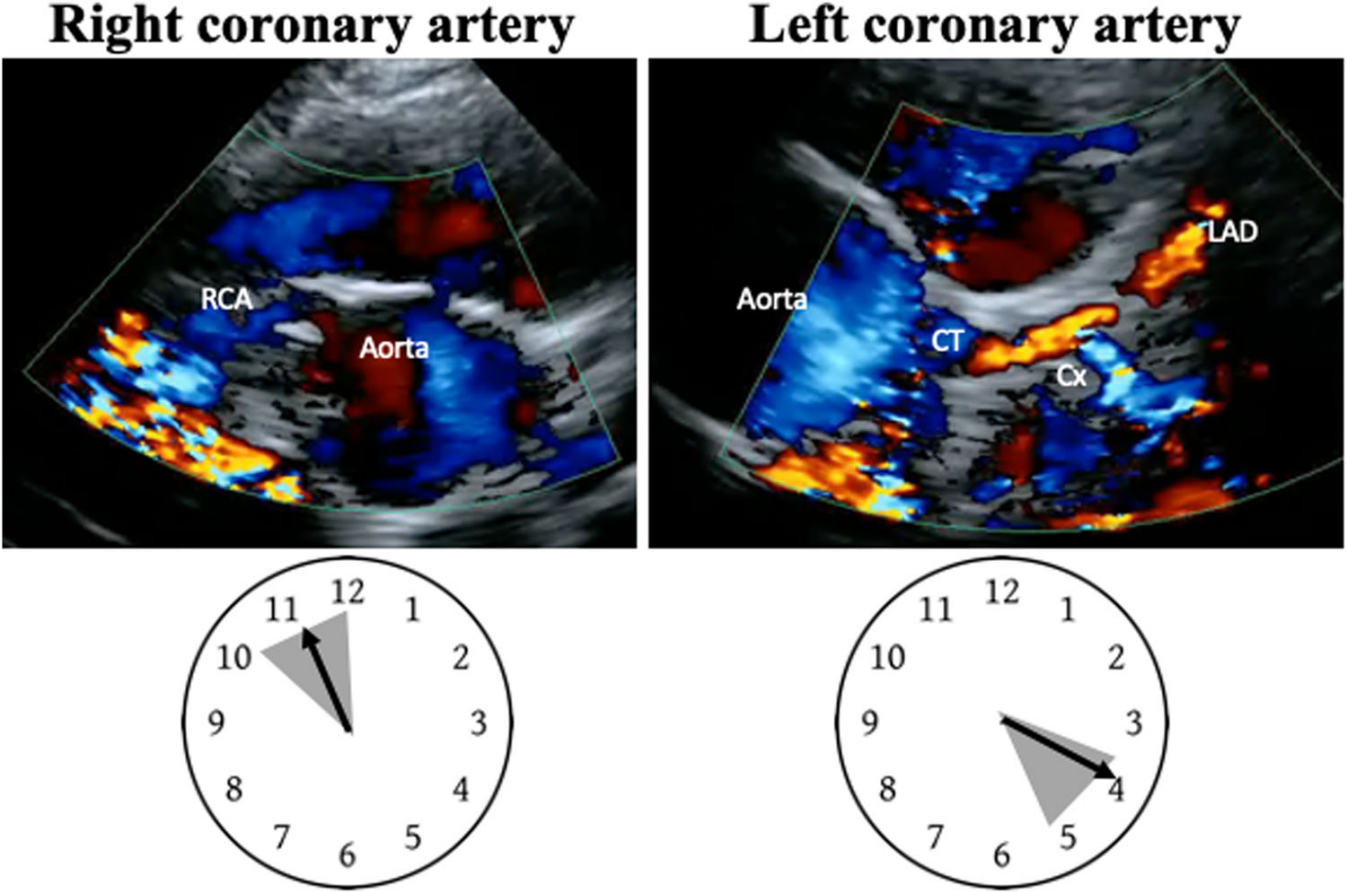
Normal position of the coronary ostia at transthoracic echocardiogram. In a clockwise fashion, the right coronary ostium is normally located at 10−12 o'clock, whilst the left coronary ostium is at 4−5 o'clock. The gray areas in the clock represent the normal position where the coronary ostia can be imaged. The color Doppler flow can be very helpful in detecting the first tracts of the coronary arteries. In the left panel, the right coronary artery appears in blue color and the common trunk is visualized with a first tract in blue and, after a smooth curve, in red color. The circumflex artery is originating from the common trunk and is visualized in light blue color. CT, common trunk; Cx, circumflex coronary artery; LAD, left anterior descending artery; RCA, right coronary artery.
